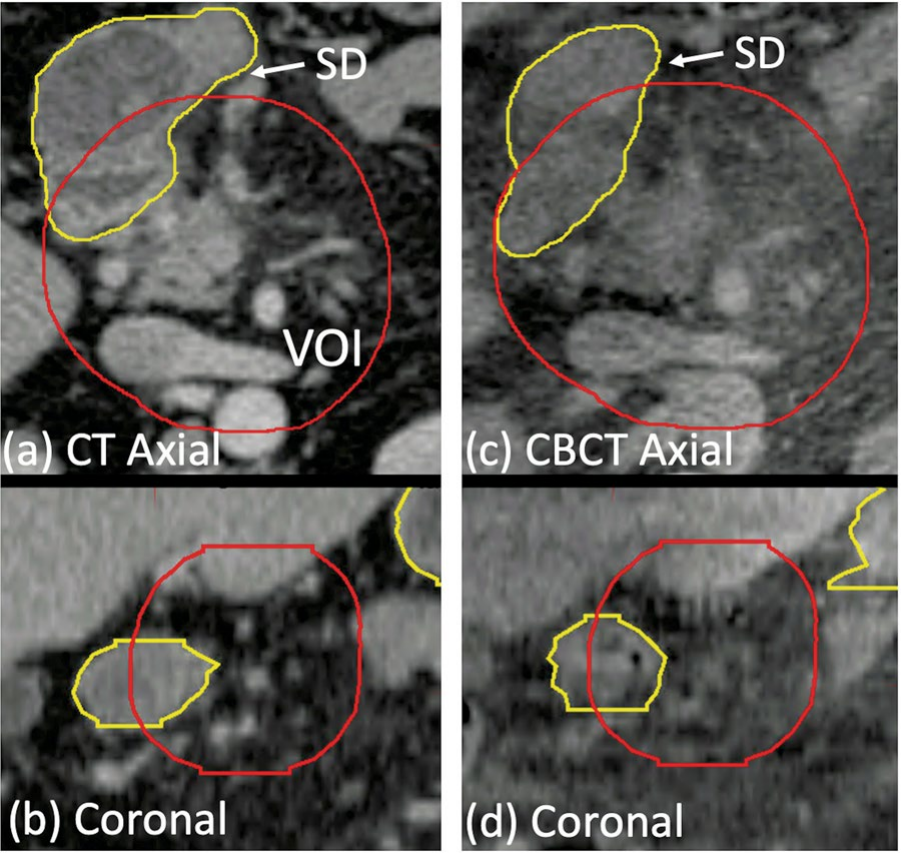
Example of physician-drawn stomach/duodenum (SD) contours on (a) axial and (b) coronal views of the planning CT, and on corresponding views (c), (d) of the CBCT scan. The volume of interest (VOI) is also outlined.
